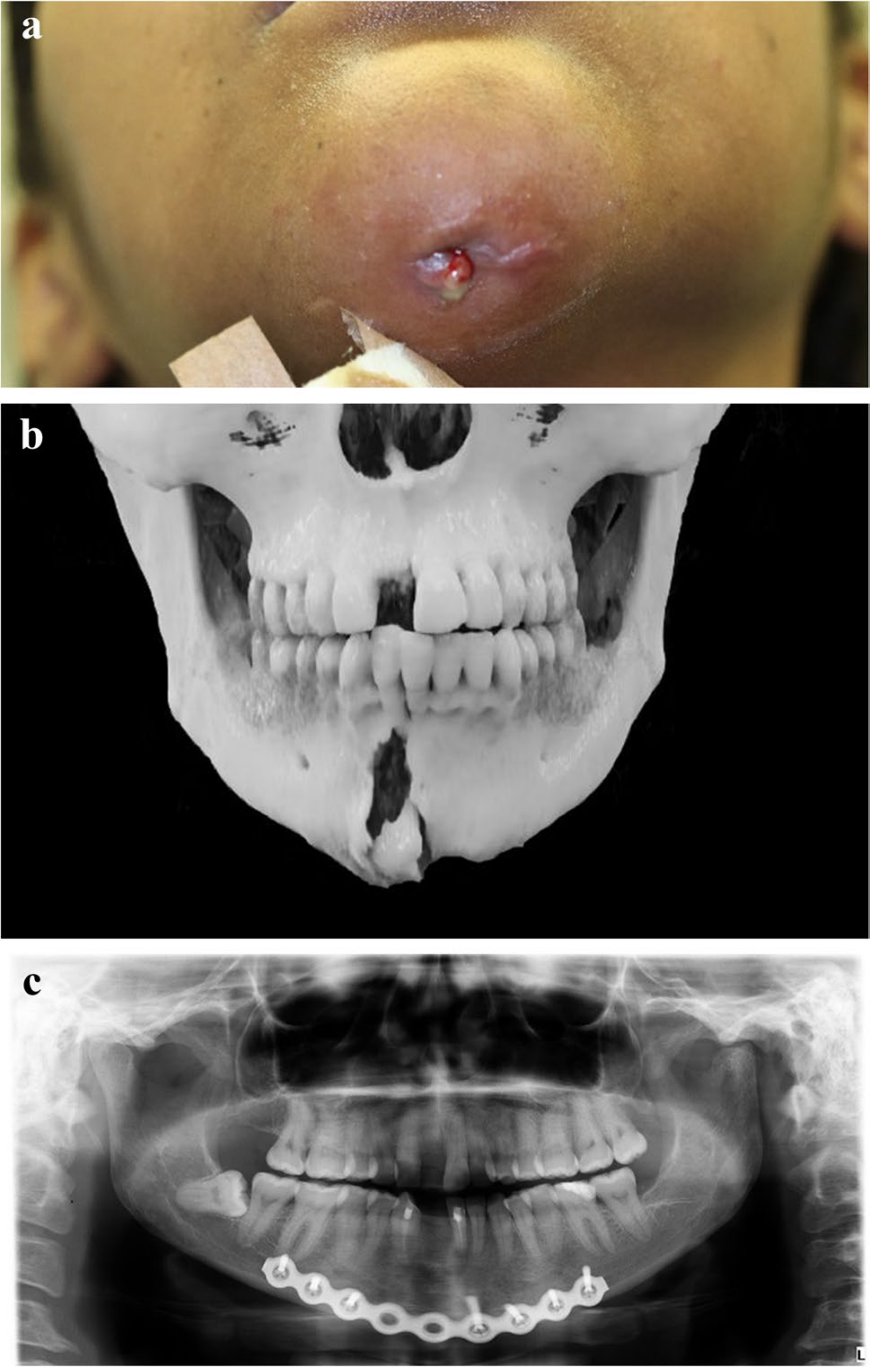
a The patient was evaluated for an infected mandibular fracture two months after falling down stairs. A purulent wound formed in the lower jaw after falling, which was treated by medical doctors with two courses of antibiotics. Before ending up in the oral and maxillofacial emergency clinic, the patient also received a blow to her mandible. The patient had an infected symphysis fracture and a fracture in the left condyle. b The patient had osteolysis, fragmentation, and chronic infection in the fracture line in the symphysis that can be seen in the preoperative 3D reconstruction of computed tomography. The occlusion was surprisingly in place, but clinically the fracture fragments were mobile, and the symphysis fracture was found to be non-ossified. c A reconstruction plate was pre-bent on a 3D-printed model to ensure the comprehensive fit of the reconstruction plate on the lower border of the mandible. Because there were no changes in occlusion, preoperative virtual reduction of the fracture was not required. Extraoral approach was used for osteosynthesis. The reconstruction plate was first fixed in place and removed during careful local debridement of the fracture line. A free iliac crest bone graft was harvested and placed in the area of ​​the mandibular bone defect. Right lower incisors were extracted during the surgery. Teeth that were close to the fracture line required endodontic treatment. Patient developed recurrent infection five weeks postoperatively, which, however, did not affect the ossification process. Patient’s (Fig. 2a) dental panoramic radiograph confirms a favorable recovery process 4.5 months postoperatively. Left condyle fracture healed without surgery
